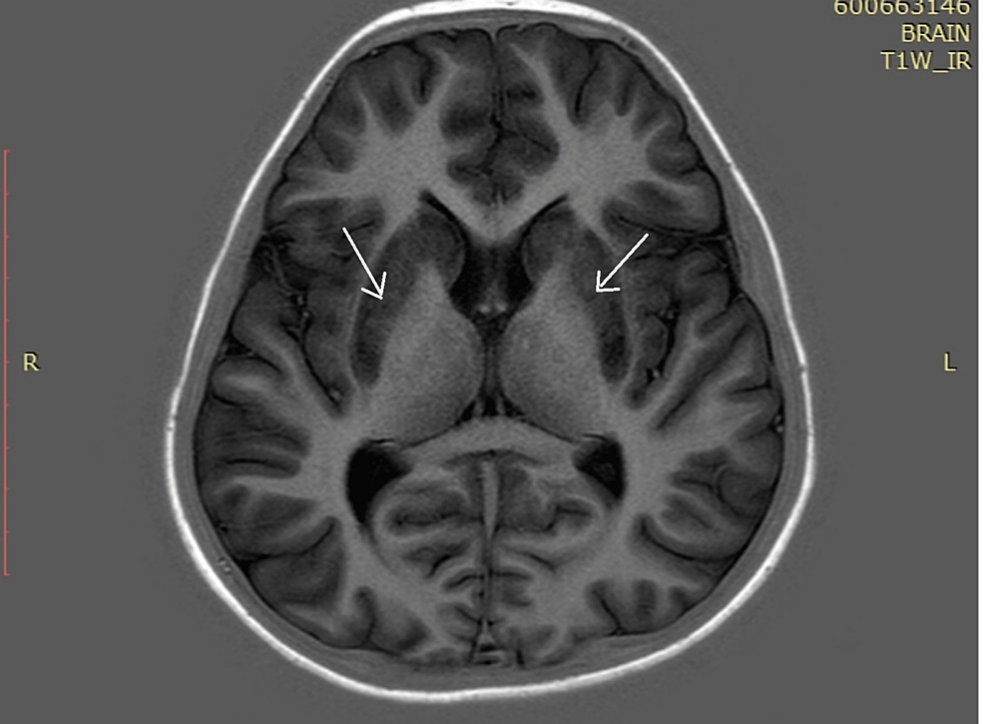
MRI T1-weighted sequence showing diffuse and symmetric hyperintensity of bilateral caudate and lentiform nuclei MRI: Magnetic Resonance Imaging
